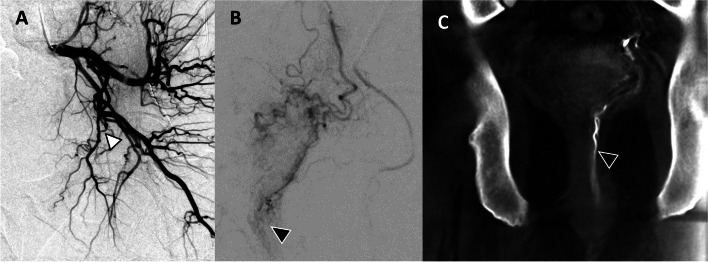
Left prostatic artery identified arising off the left internal pudendal artery (white arrowhead). B Selective DSA from the left prostatic artery shows a possible rectal supply from the capsular branch of the prostatic artery (black arrowhead). C This is confirmed on CBCT (black arrowhead). Subsequently the rectal branch was coiled before safe embolization of the prostate
